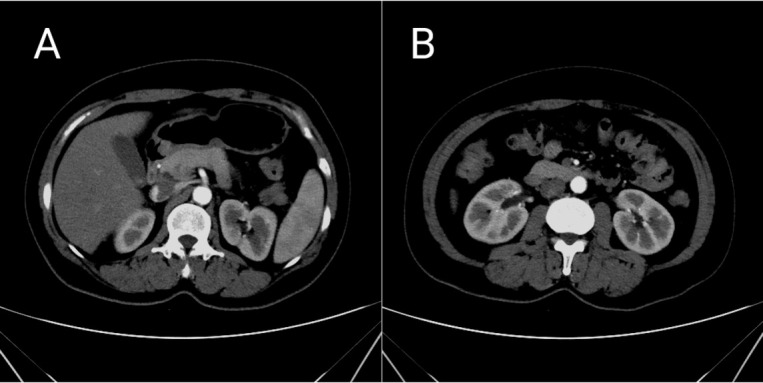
CT Abdomen with IV Contrast The coronal image of contrast-enhanced CT scan revealed that the lesion was within normal limits, and the gastric wall was poor filling. On transverse, the intestinal tract is normal. There was no sign of ileus. CT= Computed Tomography; IV= intravenous
